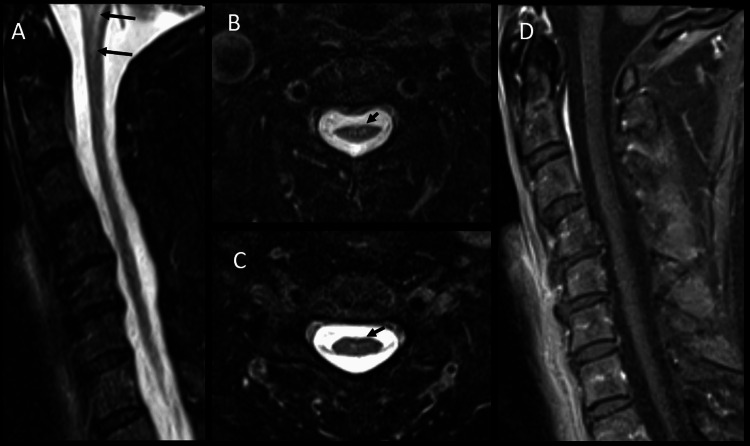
Cervical spine MRI Sagittal T2-STIR (A) and axial T2-STIR at the level of C3 (B) and C5 (C) demonstrate abnormal T2 signal involving the medulla and upper cervical spinal cord at the level of C1 (black arrows on A) and central cord (black arrows on B and C) with mild diffuse cord atrophy. There is no abnormal enhancement within the cord on post-contrast sag T1 fat-saturated imaging (D). STIR: short tau inversion recovery
